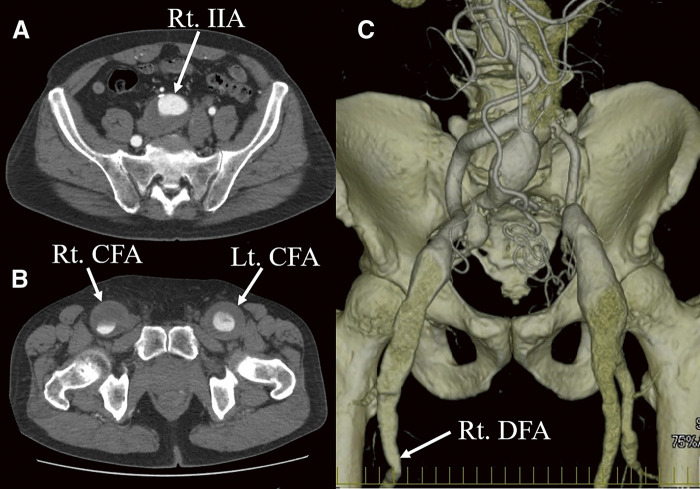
Fig. 1 Preoperative CT findings. Axial images of the abdominal CT show aneurysms of the right internal iliac artery (A) and both common femoral arteries (B), each associated with mural thrombus. Only the right deep femoral artery is opacified (C) with occlusion of the right superficial femoral artery. IIA: internal iliac artery; CFA: common femoral artery; CT: computed tomography; DFA: deep femoral artery
